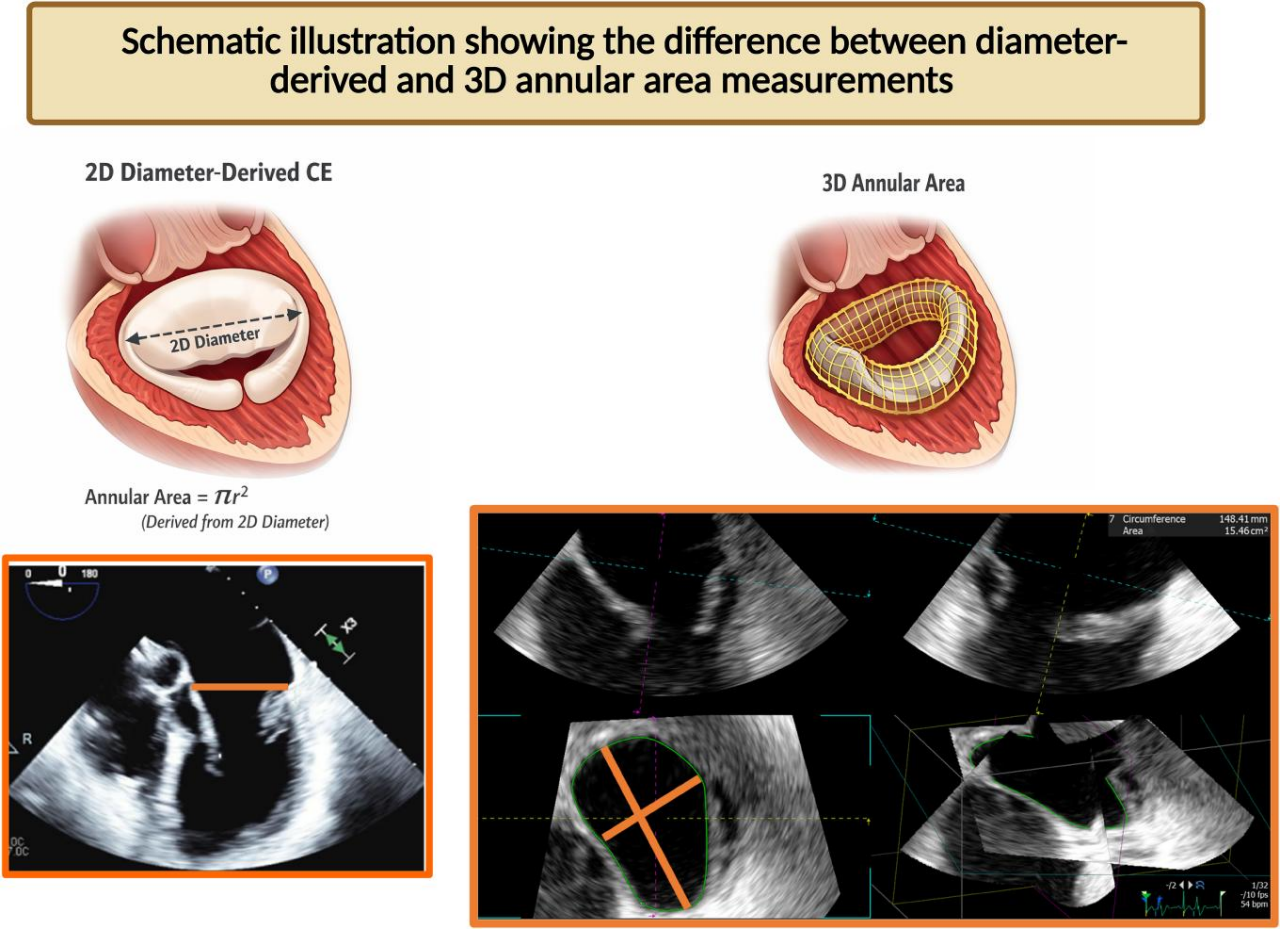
Representative case showing perfect agreement in mitral regurgitation quantification between 3D continuity equation and cardiac MRI.
